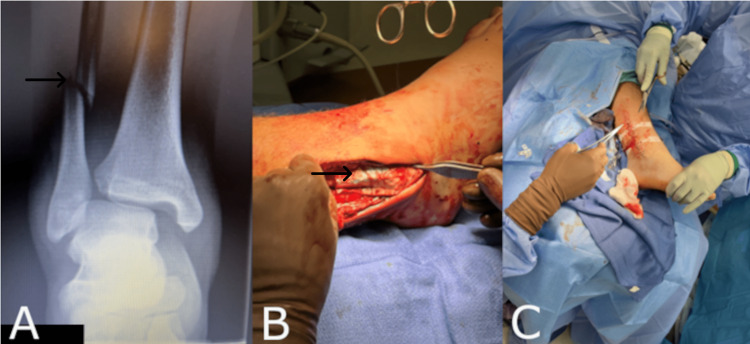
(A) Radiographic evidence of the fracture. (B) Application of the synthetic hybrid-scale fiber matrix to the surgical wound bed. (C) The surgical wound sutured closed.
